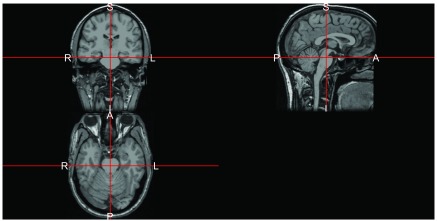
Plot of T1-weighted image from bert subject in Freesurfer after re-orientation to RPI orientation. Note, the letters denote the orientation of right/left (R/L), posterior/anterior (P/A), inferior/superior (I/S).
